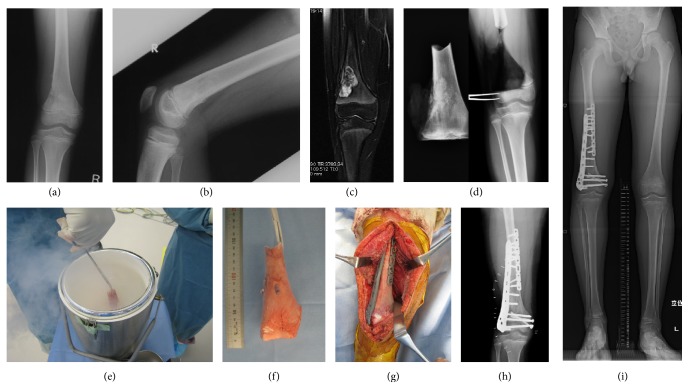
Case presentation representing a ten-year-old boy at time of surgery with osteosarcoma distal right femur. (a, b) Anteroposterior and lateral view of distal femur XR showing the tumor mass. (c) T2 weighed MRI image of the distal femur showing the tumor mass with high signal intensity. (d) Intraoperative XR showing the freely resected segment to be frozen and the host bone. (e) Intraoperative photo of free freezing. (f) The tumor bearing bone after freezing. (g) The frozen segment after repositioning and fixation. (h) Postoperative XR of the distal femur after freezing and fixation. (i) Long length film showing a LLD of 3 cm.
